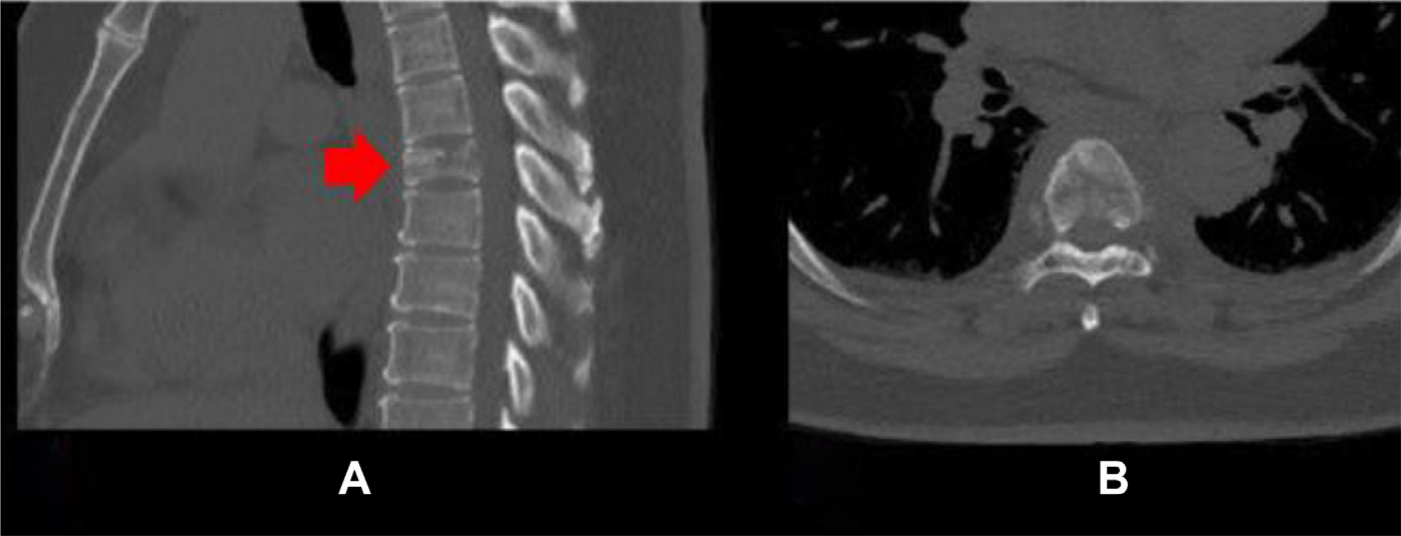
Selected thoracic region sagittal (A) and axial (B) images of computed tomography scan -bone window- revealed T6 vertebral body partial collapse with suspicion of an underlying bone lesion (red arrow).
